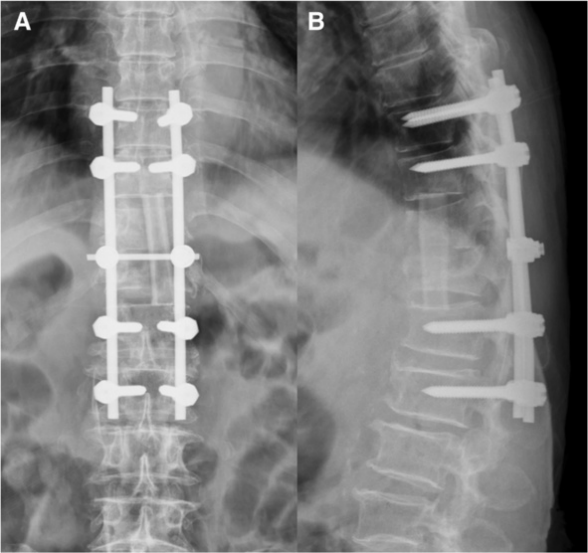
Postoperative radiographs. The postoperative anteroposterior (A) and lateral (B) radiographs revealed that the dislodged bone cement had been removed. Good correction of spinal alignment was achieved by fibular allograft implantation and posterior pedicle screw fixation.
